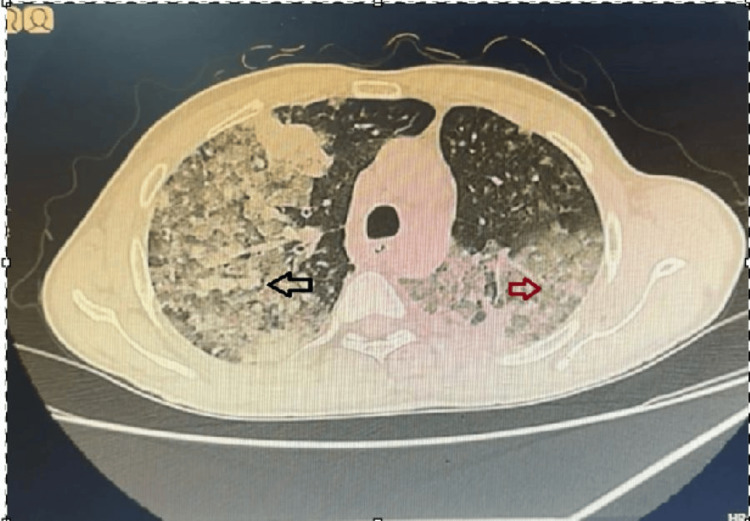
High-resolution computed tomography (HRCT) of the thorax showing dense consolidation with air bronchogram in the left lower lobe, associated with centrilobular and perilymphatic nodules.
